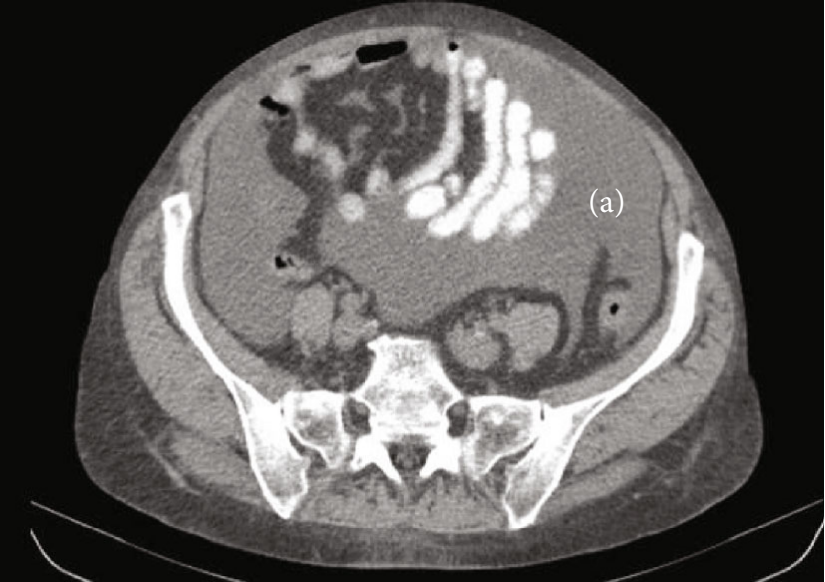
Abdominal computed tomography showing a large quantity of ascites (a).
